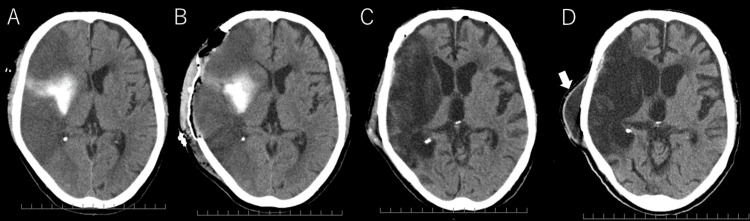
Head CT changes over time (A) CT scan on day 2 showing extensive cerebral infarction in the right middle cerebral artery territory, ventricular deformation, and midline shift. (B) Postoperative CT scan showing the status after decompressive craniectomy. (C) CT on day 117 revealing post-cranioplasty. (D) Enlarged ventricles and subcutaneous cerebrospinal fluid collection (arrow) are also noted. CT, computed tomography
